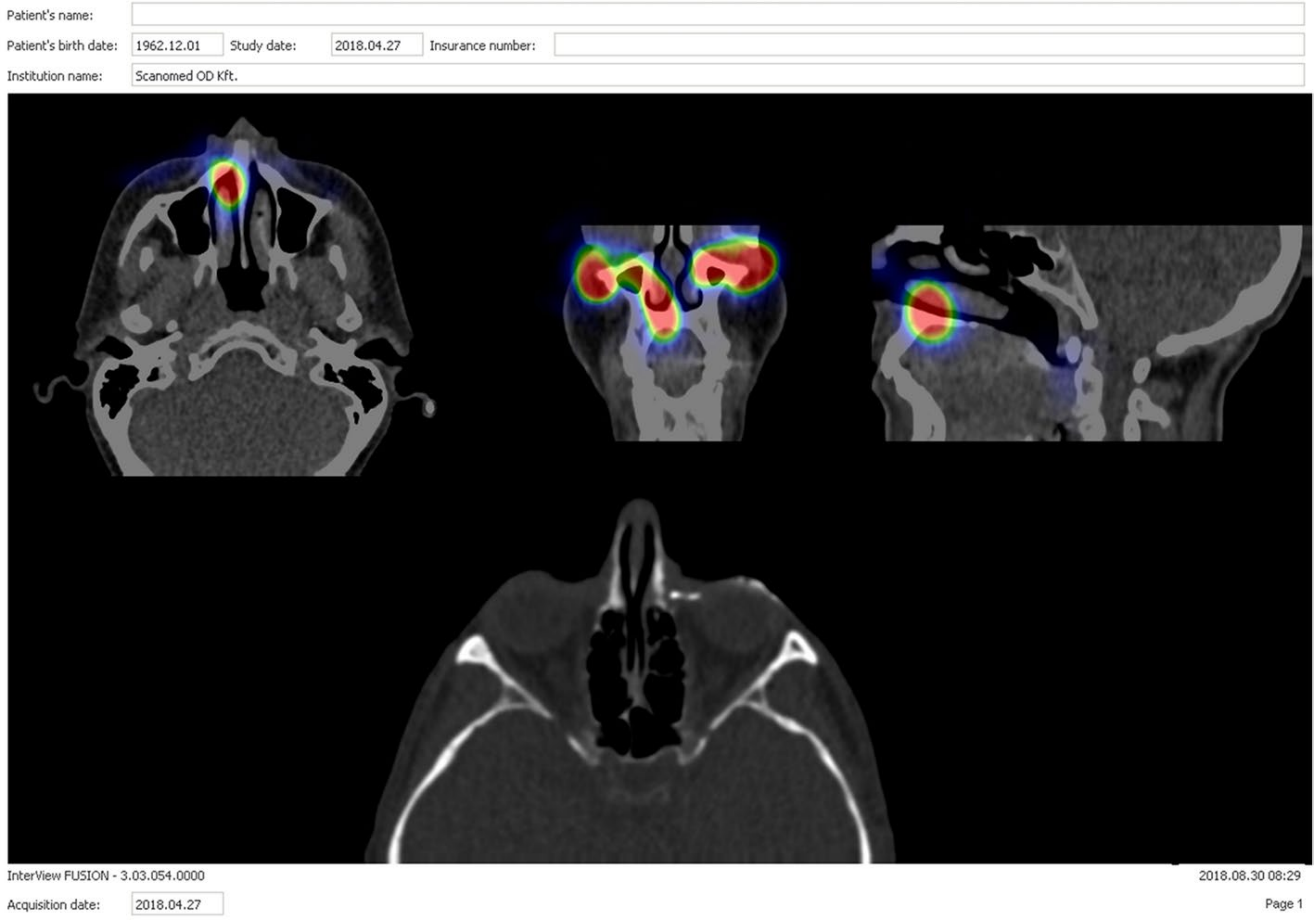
SPECT/CT result of the patient represented in Fig. 1 on coronal and sagittal images: activity of ^99m^Tc-sodium pertechnetate can be detected on the right region of the nasolacrimal duct, but there is no outflow on the left side. *SPECT*/*CT* single-photon emission computed tomography/computed tomography
